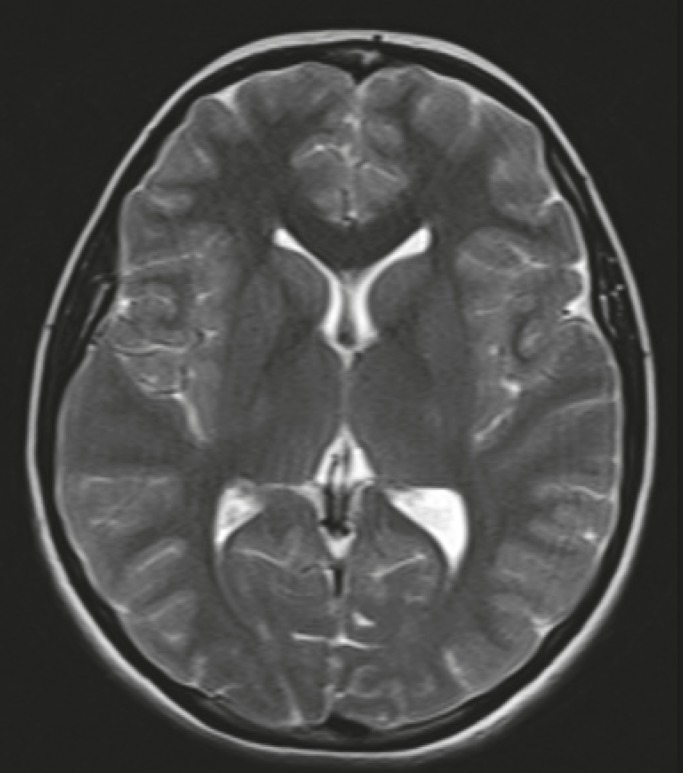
MRI showed no cerebral abnormality
